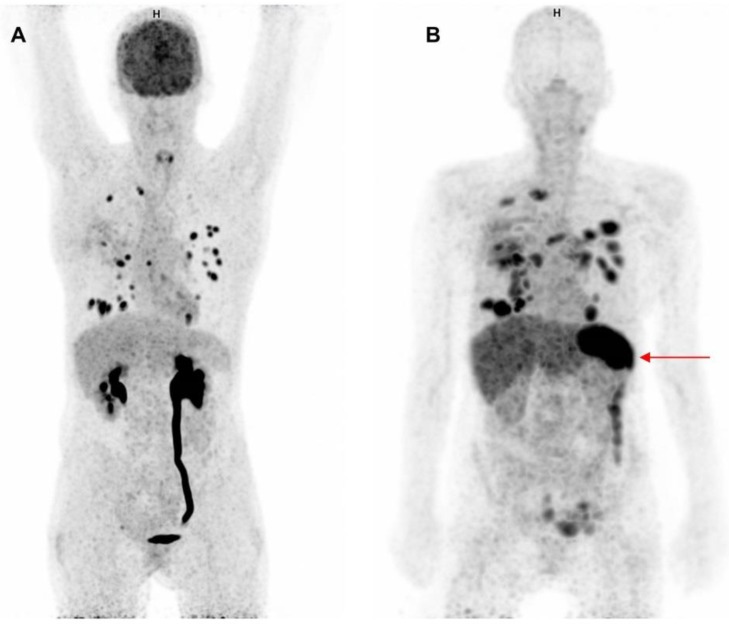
Comparison of ^18^F-FDG-PET and ^89^Zr-atezolizumab-PET imaging. Maximum intensity projections of ^18^F-FDG-PET, 1 h after tracer injection (A) and ^89^Zr-atezolizumab-PET, 7 days after tracer injection (B) of a 53 year old woman diagnosed with NSCLC. Both imaging modalities show uptake in multiple intra-pulmonary metastases. The ^18^F-FDG-PET scan shows physiological high uptake in the brain and excretion via the kidneys. At the moment of this scan the patient had a post-renal obstruction due to a kidney stone. The ^89^Zr-atezolizumab-PET scan shows high uptake in the spleen (red arrow). The ^18^F-FDG-PET scan was performed 46 days prior to the ^89^Zr-atezolizumab-PET scan. Both scans were scaled equally (0-8).
